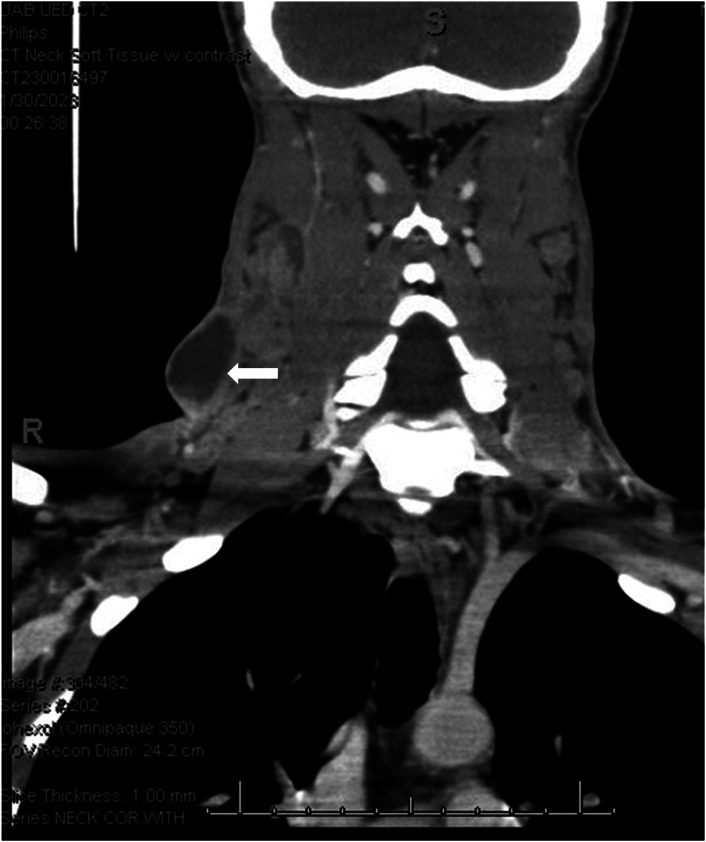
Computed tomography of the neck with intravenous contrast revealed multiple necrotic and cystic-appearing lymph nodes (arrow).
